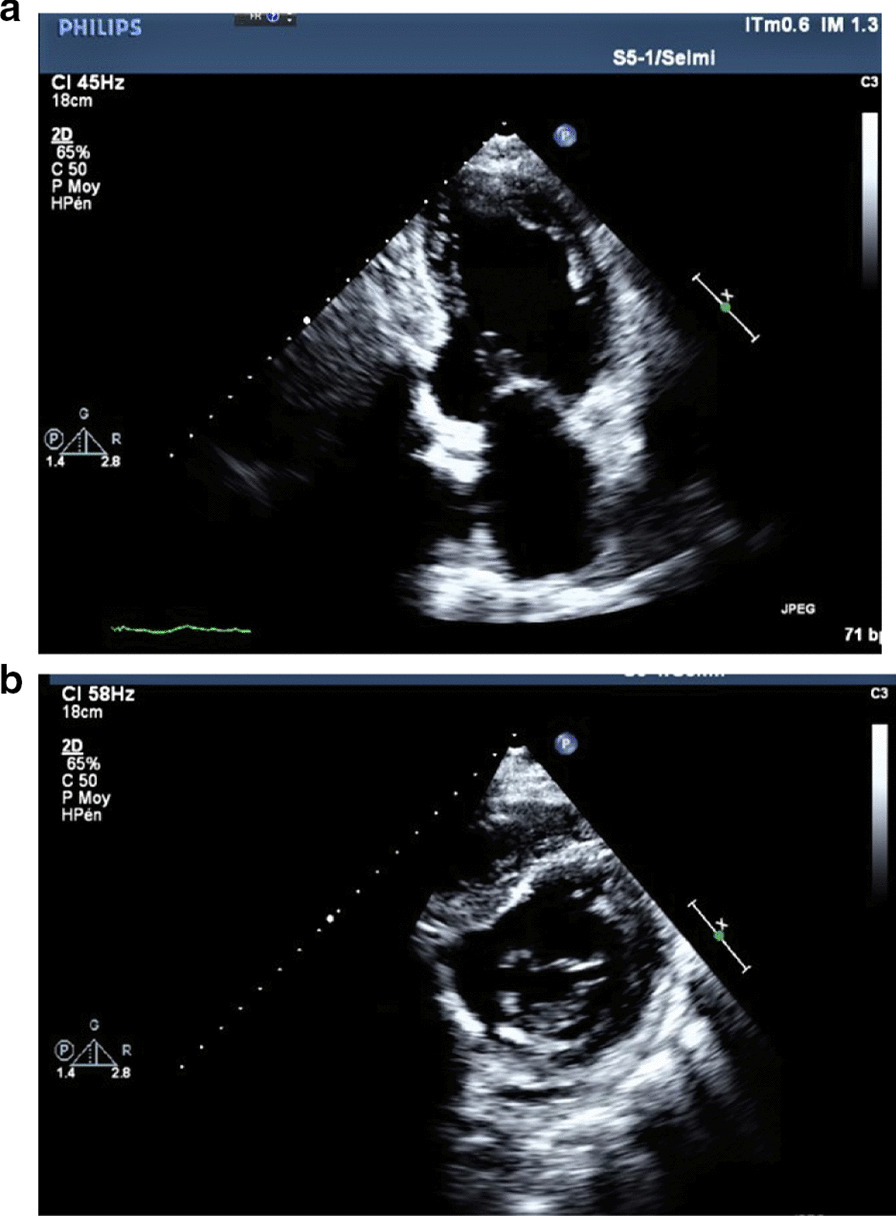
a Postoperative transthoracic echocardiogram from apical two-chamber view showing ventricle restoration. b Postoperative transthoracic echocardiogram from parasternal short view showing ventricle restoration
